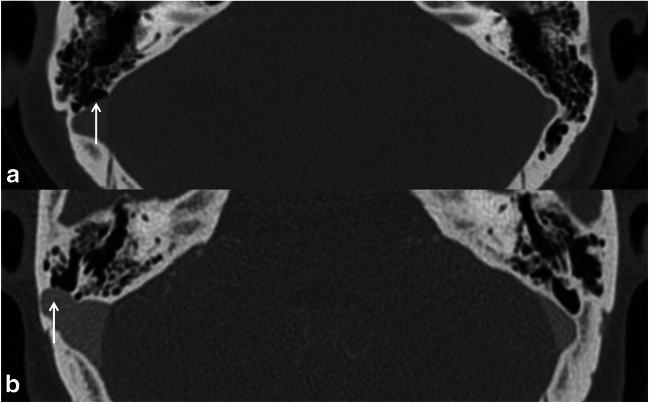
Axial high-resolution CT venography images show sigmoid sinus wall dehiscence (a) and sigmoid sinus diverticulum (b) on the right sides
